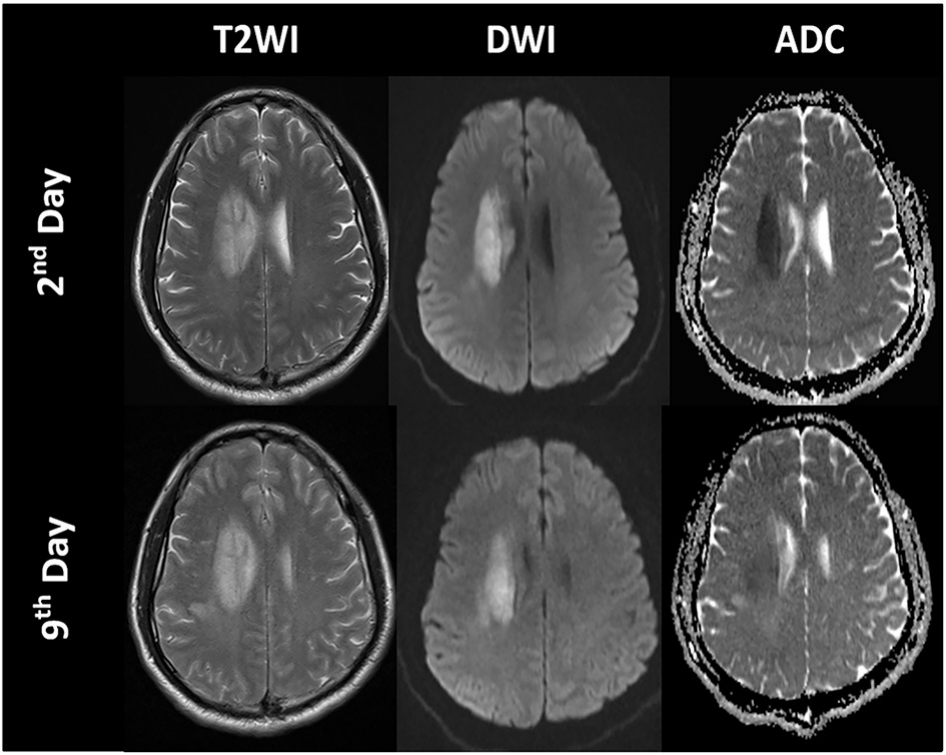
Time course of the signal intensities in T2-weighted imaging (T2WI), diffusion-weighted imaging (DWI), and apparent diffusion coefficient (ADC) maps of a patient with a right paraventricular infarct.
